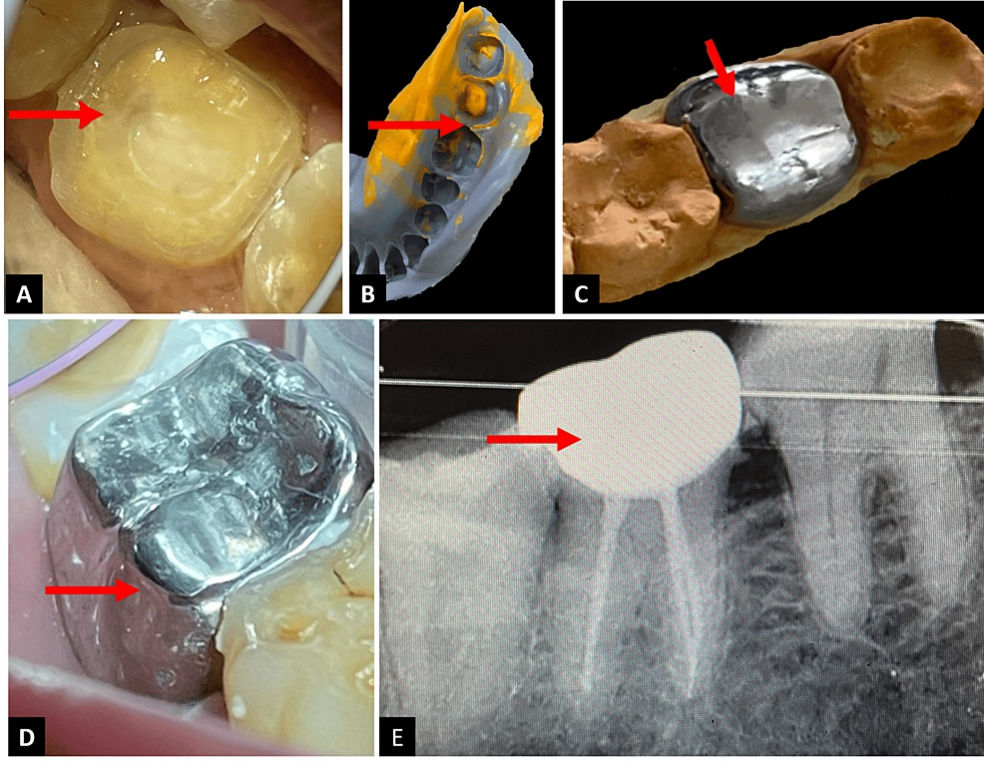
Endocrown with tooth number 47 (A) Endocrown preparation with tooth number 47. (B) Impression after endocrown preparation with tooth number 47. (C) Metal endocrown fabricated with tooth number 47. (D) Post-cementation clinical picture showing endocrown placement with tooth number 47. (E) Post-cementation radiograph showing endocrown placement with tooth number 47.
